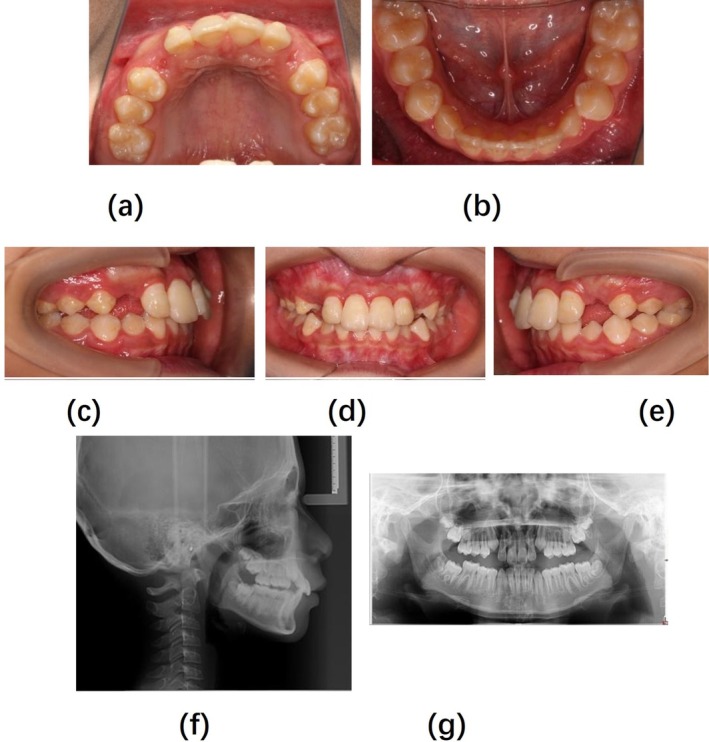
Clinical and radiograph views during orthodontic treatment. (a–e) Intraoral views during orthodontic treatment; (f–g) radiograph views during orthodontic treatment.
